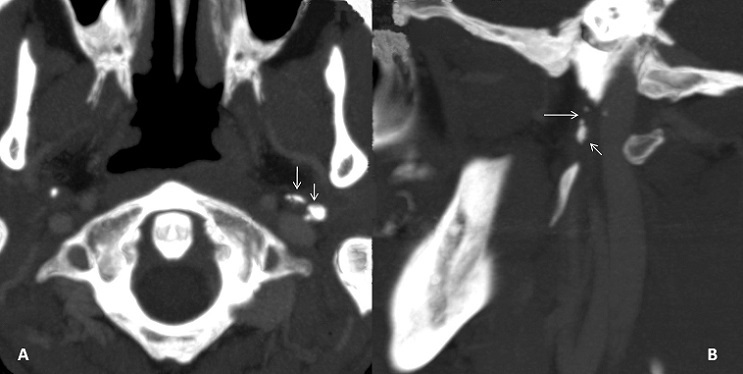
Targeted axial (Panel A) and left sagittal (Panel B) MIP reconstructed CT scan images with bone window of the neck showed a comminute fracture of the styloid process at the base of the skull (arrows)
